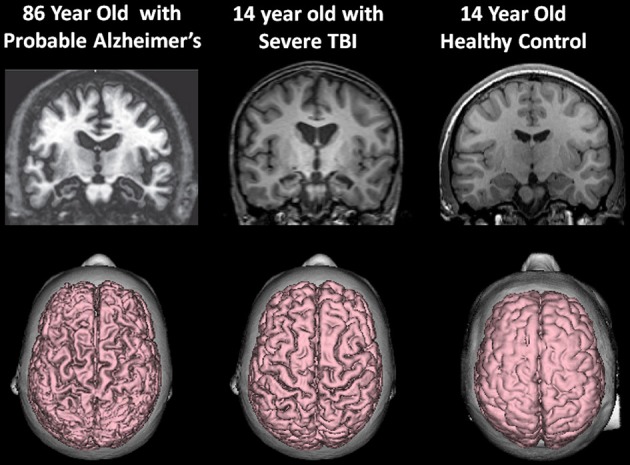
(Top) T1-weighted coronal images approximately at the same level showing hippocampal atrophy in the 86-year-old patient with a clinical diagnosis of Alzheimer's disease and the 14-year-old patient with severe traumatic brain injury (TBI). Note compared to the control coronal image on the right, both the TBI case and the Alzheimer's exhibit hippocampal atrophy, ventricular dilation and sulcal widening. (Bottom) All images are 3-D renderings from volume acquisition magnetic resonance imaging (MRI) depicting the dorsal view of the brain in each subject described above. Note the similarity of the diffuse pattern of atrophic change that has occurred in both the patient with Alzheimer's disease and the adolescent who survived severe TBI. Clearly, the elderly patient with Alzheimer's has more severe atrophy but nonetheless the atrophy in the TBI adolescent is substantial, especially when compared to the typical developing adolescent control. Note: The patient with Alzheimer's disease is taken from Jacobson et al. (2009); this patient's clinical findings, including additional neuroimaging and neuropsychological details are described in that publication. Reproduced with permission from John Wiley and Sons.
